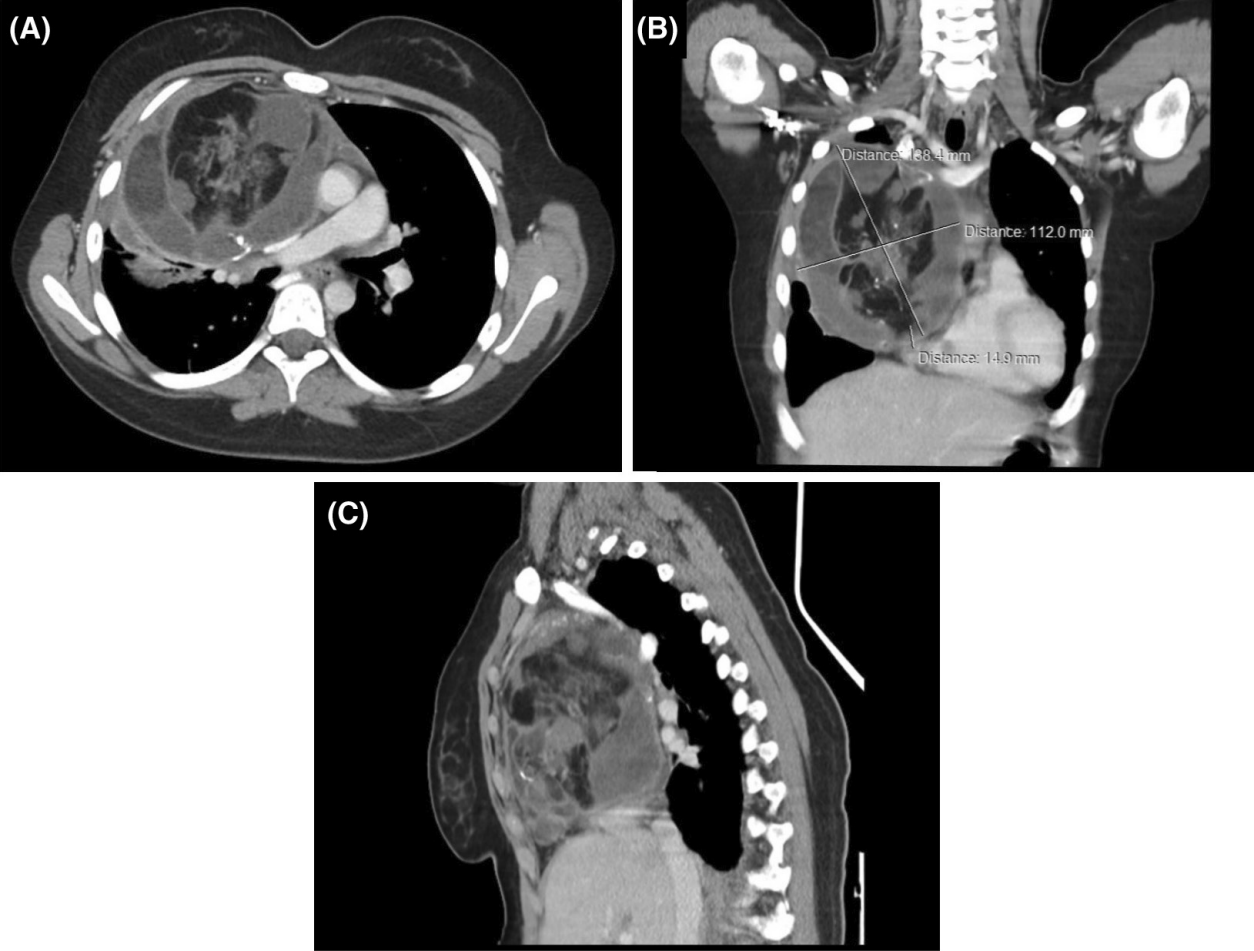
(A) Axial, (B) coronal, (C) sagittal CT section showing a well‐defined heterogeneous mass occupying the right part of the anterior mediastinum and a part of the right hemithorax, consisting of variable densities of fat, liquid, and bonny tissues.
